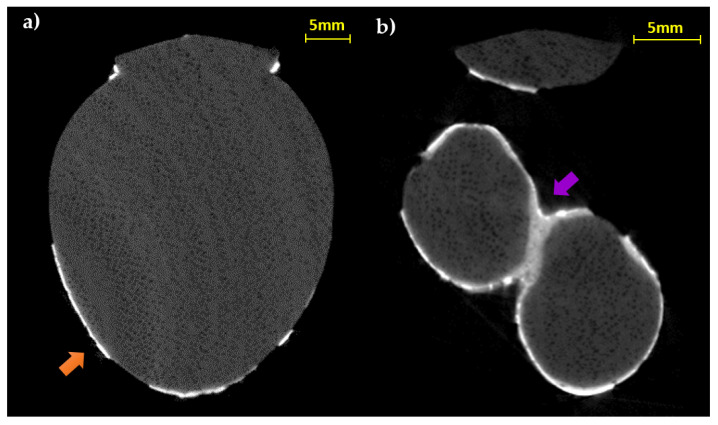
CT horizontal slices of the body (a) and the legs (b) (orange arrow: thin preparation material for the gold leaf adhesion; purple arrow: thick preparation material between the legs).
